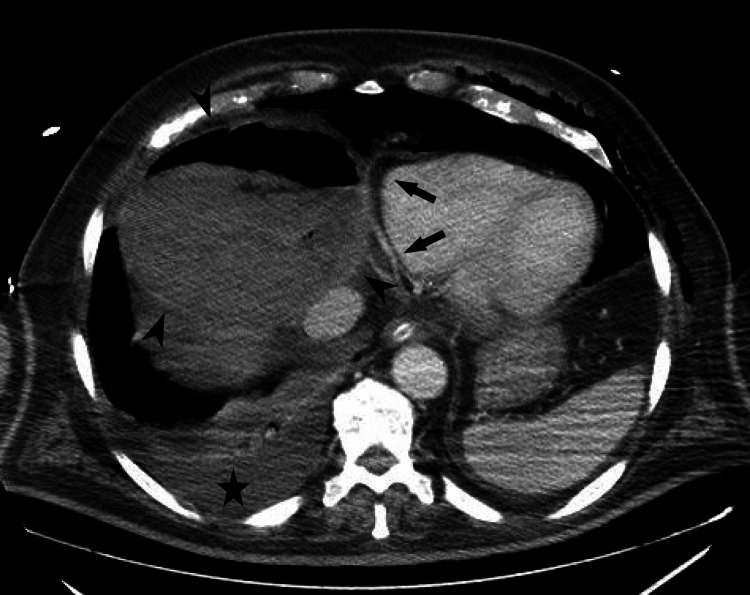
Axial CT scan showing the liver abscess (indicated by arrowheads), right pleural effusion (indicated by the star) and pericardial thickening (indicated by arrows).
